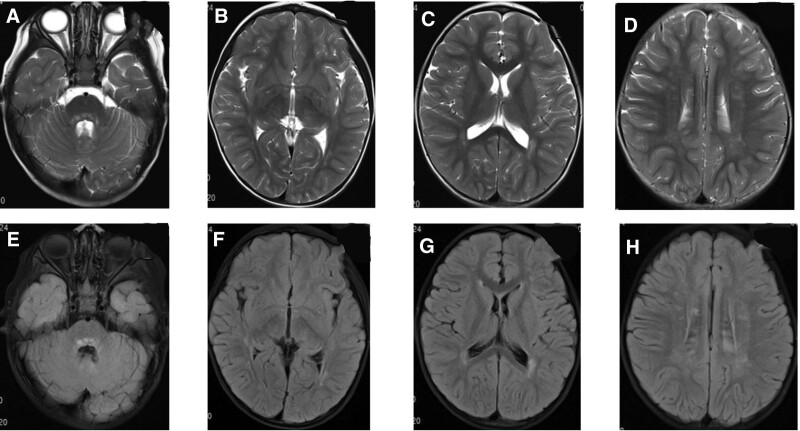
Cranial MRI of Case 1 at the age of 3 years. Multiple patchy and slightly long T1, long T2, and FLAIR high signal shadows found at white matters of bilateral frontal, parietal, and occipital lobes, as well as bilateral para-ventricular white matters, left thalamus, bilateral cerebellar hemispheres, and dorsal pons, with unclear boundaries. Some lesions showed small round shapes, some lesions were more limited than before, and some showed higher signals than before. The number of lesions in the left thalamus, dorsal pons, and bilateral lateral ventricles was higher than before. FLAIR = fluid-attenuated inversion recovery, MRI = magnetic resonance imaging.
